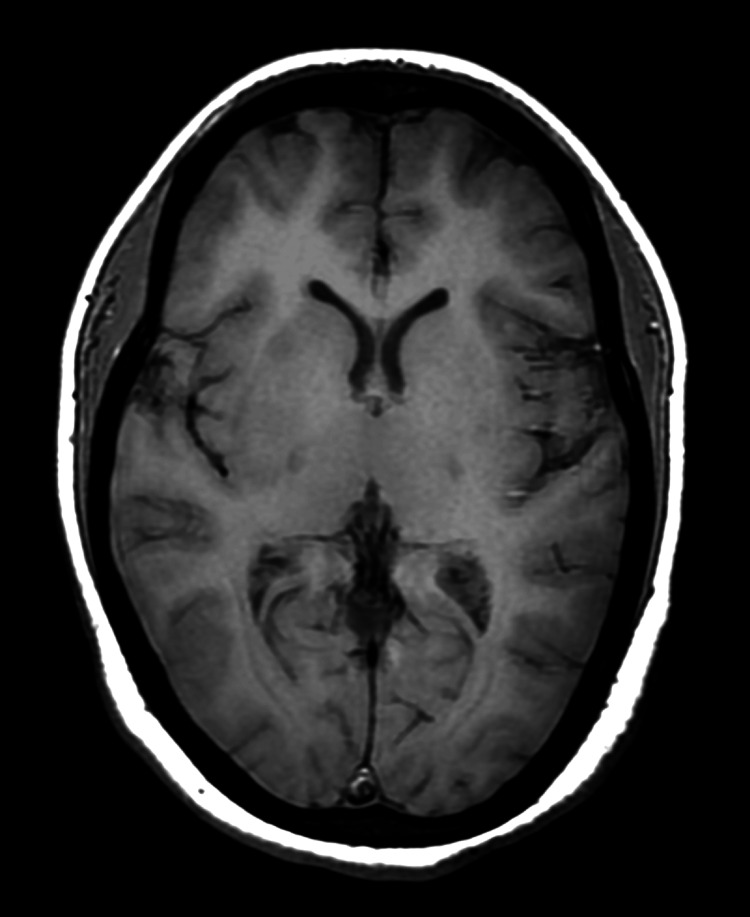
MRI brain (T1W) showing bilateral isointense signals in bilateral basal ganglia and occipital regions. MRI: magnetic resonance imaging; T1W: T1 weighted
